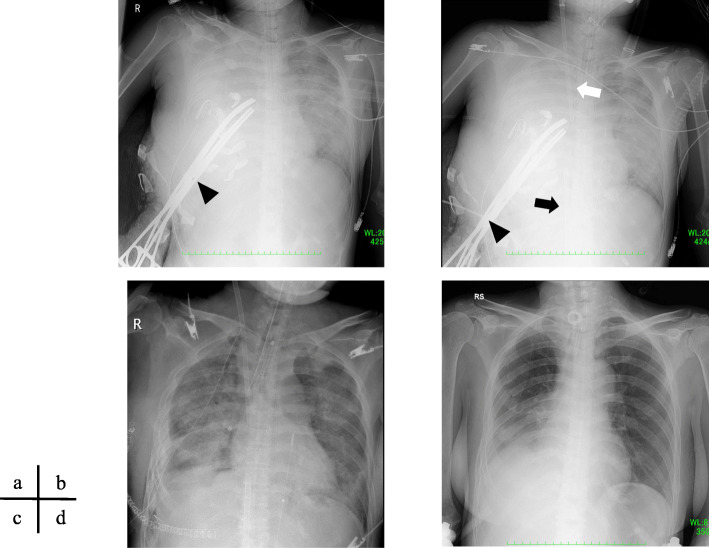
Chest X-ray findings post operation. a Chest X-ray findings following damage control surgery (thoracotomy). The bleeding source was clamped (*black arrowhead*) and intrathoracic packing was performed. b Chest X-ray findings post establishment of veno-venous extracorporeal membrane oxygenation system. The catheter to establish the veno-venous extracorporeal membrane oxygenation was placed from the right internal jugular vein (feeding catheter, *white arrow*) and right femoral vein (drainage catheter, *black arrow*). c Chest X-ray findings after planned reoperation on day 2. d Chest X-ray findings on day 15 showed a significant improvement in both lung areas
